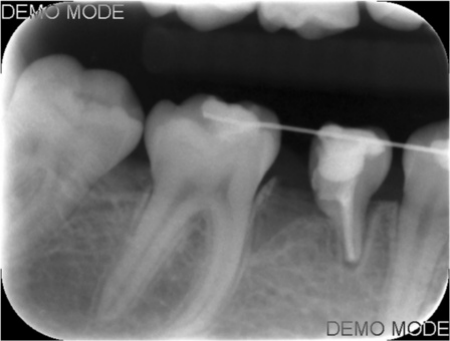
Radiograph 10 weeks post replantation showing the absence of periodontal ligament and root ankylosis
